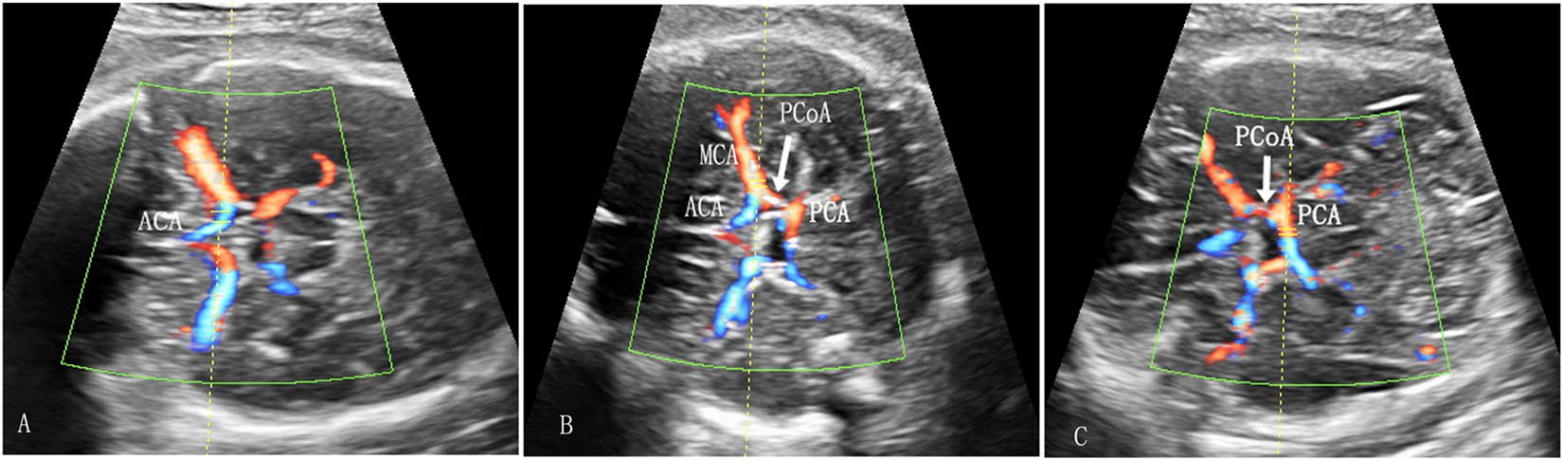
The fetal brain circulation was examined by color Doppler imaging in an axial plane at the level of the circle of Willis. The ACA was recorded with the sample volume placed between its origin from the internal carotid artery and the anterior communicating artery (A). The MCA was recorded at the proximal segment after its origin from the circle of Willis (B). The PCA was recorded with the sample volume placed between its origin from the basilar artery and the posterior communicating artery (C). ACA, anterior cerebral artery; ACoA, anterior communicating artery; MCA, middle cerebral artery; PCA, posterior cerebral artery; PCoA, posterior communicating artery.
